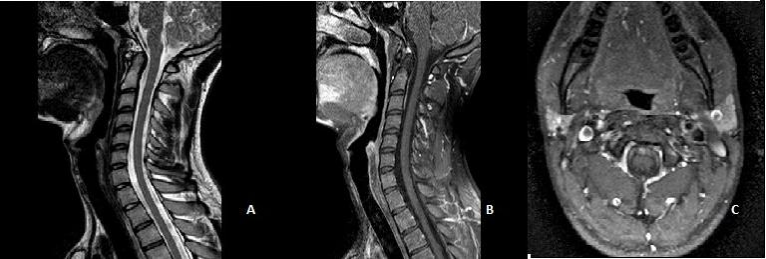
After six months cervical MRI showed complete regression of meningioma in T2 weighted (A), T1 weighted sagittal (B) and axial (C) images with lineer contrast enchancement without any solid lesion*.
